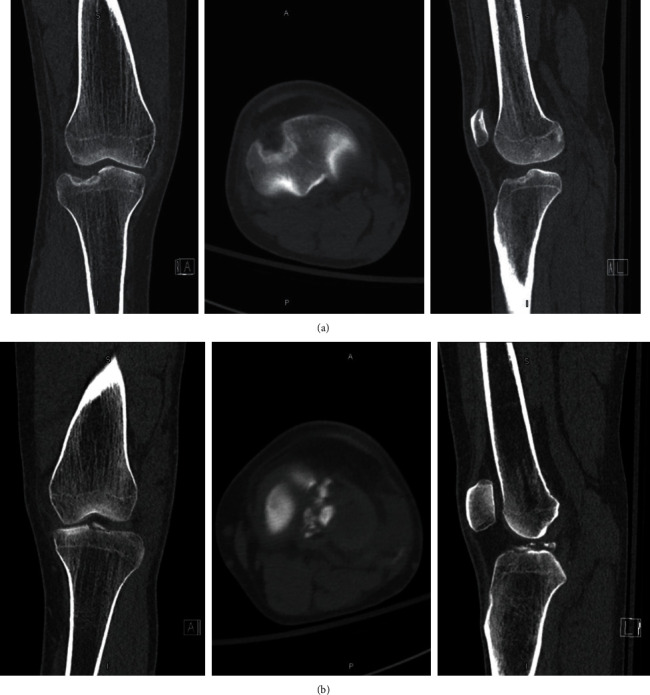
(a) CT scan of the right knee depicting a lateral tibial plateau fracture. (b) CT scan of the left knee depicting a tibial spine fracture.
